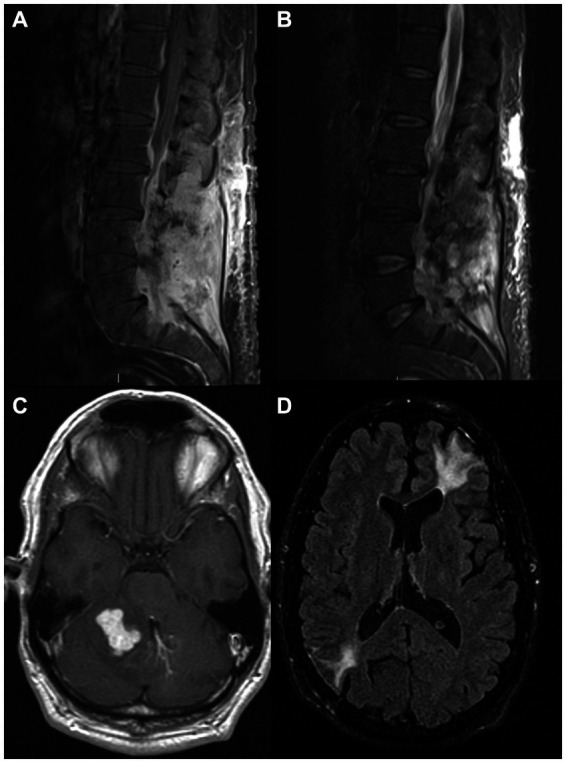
MRI images from two patients with neurological manifestations of nontuberculous mycobacteria. MRI images from a 56-year-old man with *Mycobacterium abscessus* isolated from a lumbosacral epidural abscess with sacral osteomyelitis and arachnoiditis [(A) – postcontrast T1, (B)– STIR], and a 39-year-old man with sarcoidosis and IRF-8 variant with a cerebellar enhancing lesion at presentation (C) and an encephalomalacia years after resection of left frontal and right parietal lesions due to *Mycobacterium avium* complex (D). IRF8, Interferon Regulatory Factor 8; STIR, short tau inversion recovery.
